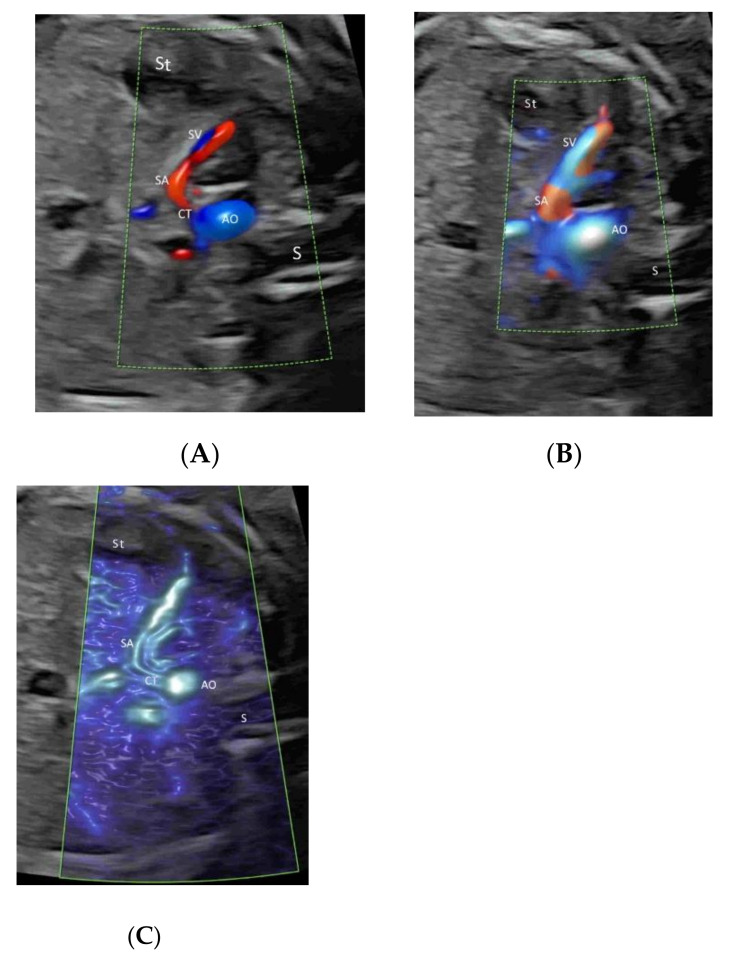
Color Doppler (A), high-definition (B) and microvascular (C) flow imaging in a transverse plane of a normal fetus at 23 weeks’ gestation showing the splenic artery (SA), a branch of the celiac trunk (CT) arising from the aorta (AO). St, stomach; S, spine.
